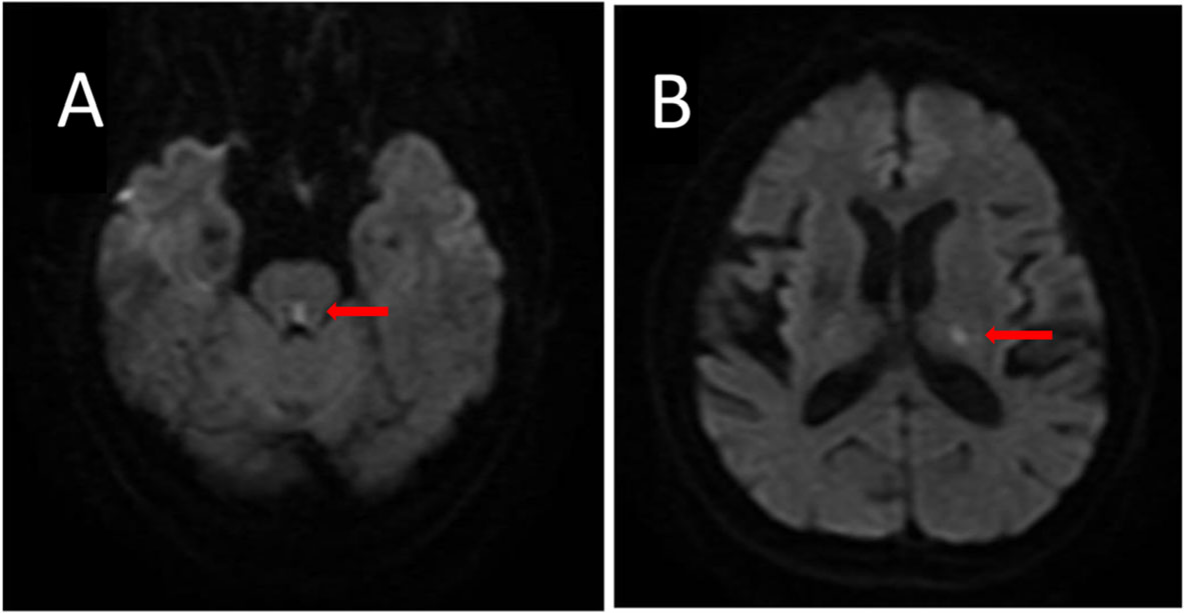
Diffusion-weighted brain magnetic resonance imaging shows two infarctions in bilateral dorsomedial pons (red arrow in a) and left thalamus infarction (red arrow in b)
